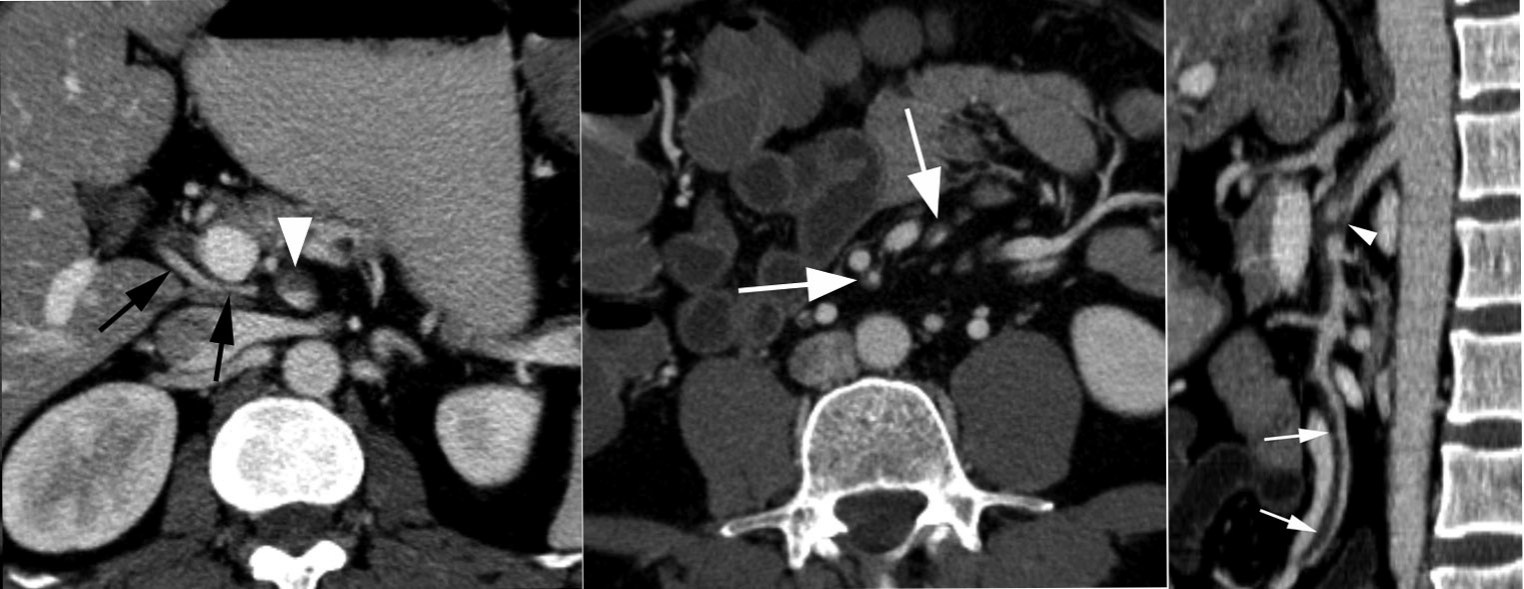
Contrast-enhanced computed tomography during the portal phase. (A) The axial image at the level of the proximal superior mesenteric artery shows a dissection with a mural thrombus (white arrowhead), which is associated with minimal inflammation of the mesentery. Note the accessory right hepatic artery (black arrows) that runs behind the portal vein. (B) The axial image at a lower level shows extension of the dissection to distal arterial branches (white arrows). The small bowel and the colon have a normal appearance. (C) The curved multi-planar reconstruction along the main trunk of the superior mesenteric artery shows the origin of the dissection approximately 1 cm from its ostium and distal extension to a jejunal artery (white arrows). The true lumen of the superior mesenteric artery is severely compressed by a dilated, partially thrombosed false lumen (white arrowhead).
